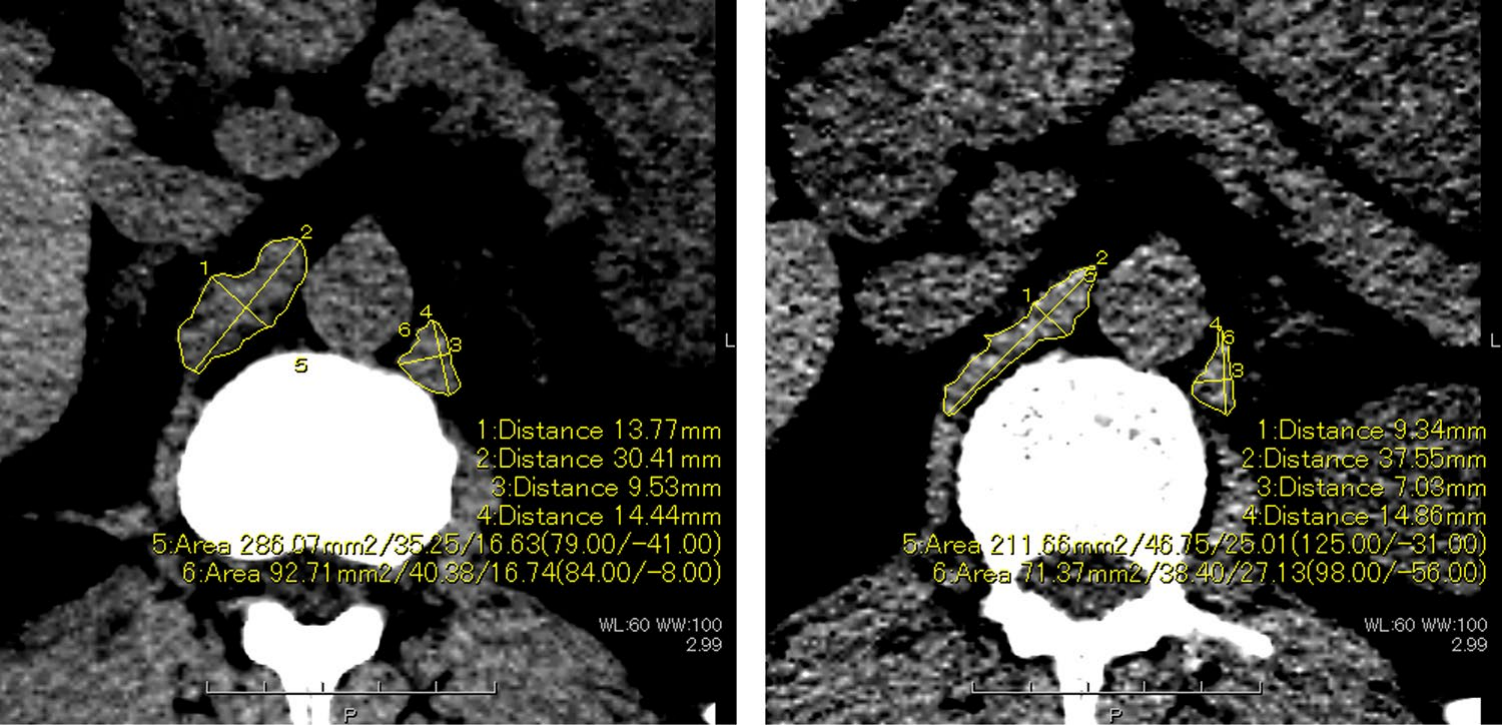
Measurements of the diaphragmatic crura at inspiratory and expiratory CT using a commercially available interpretation viewer. The left figure presents the cross-sectional image at inspiration, and the right figure presents the cross-sectional image at expiration at the orifice of the superior mesenteric artery under the mediastinal window
